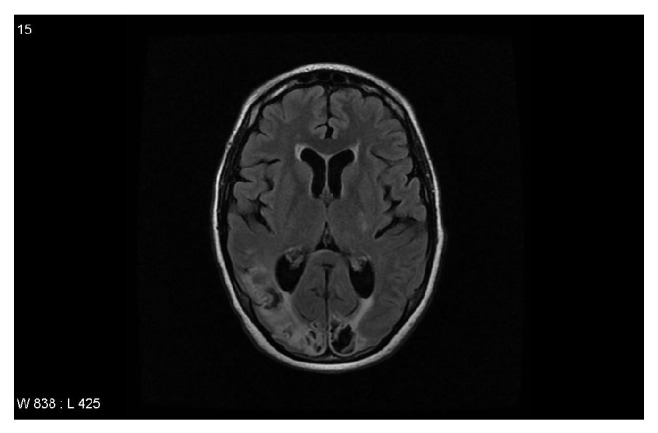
MRI brain 5 months after presentation.
